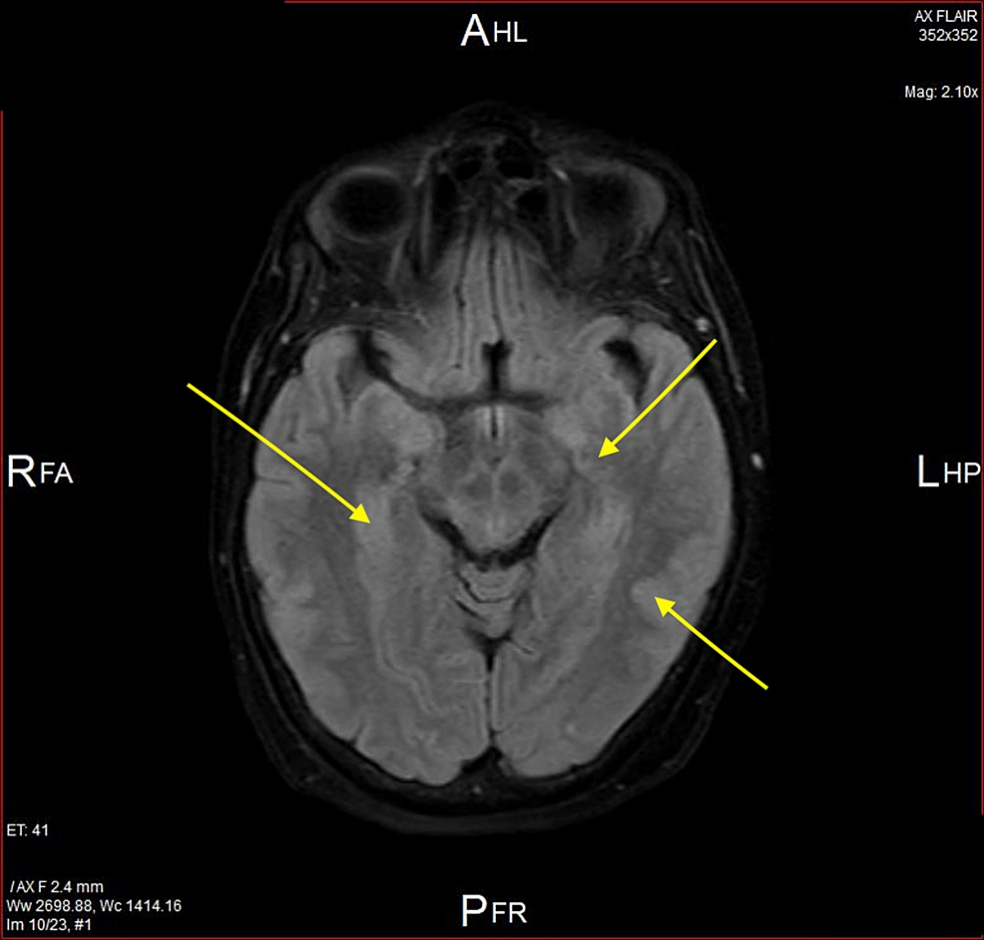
Initial magnetic resonance image (MRI) showing multifocal patchy T2/FLAIR signal abnormalities Yellow arrows in the image indicate multiple hypodense regions. FLAIR, Fluid-attenuated inversion recovery.
